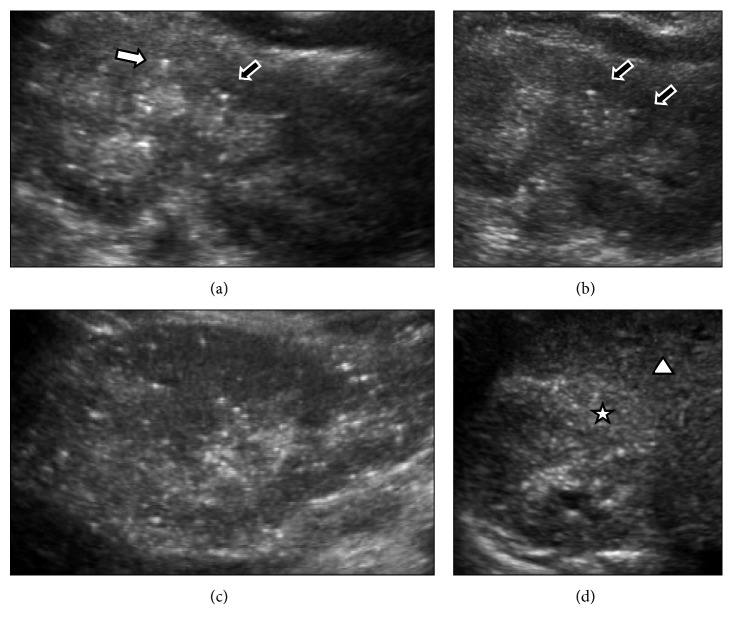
Renal ultrasound. (a) Sagittal left kidney: echogenic pyramids, punctuate hyperechoic foci in cortex, comet tail artifact (black arrow outlined in white), and small cyst with through transmission (white arrow with black outline). (b) Sagittal left kidney: echogenic pyramids with many punctuate hyperechogenicities. Two tiny sonolucent cysts with through transmission (black arrows with white outlines). (c) Sagittal right kidney: many punctuate cortical hyperechogenicities. (d) Transverse right kidney: generally increased cortical echogenicity (star) relative to adjacent liver (triangle).
